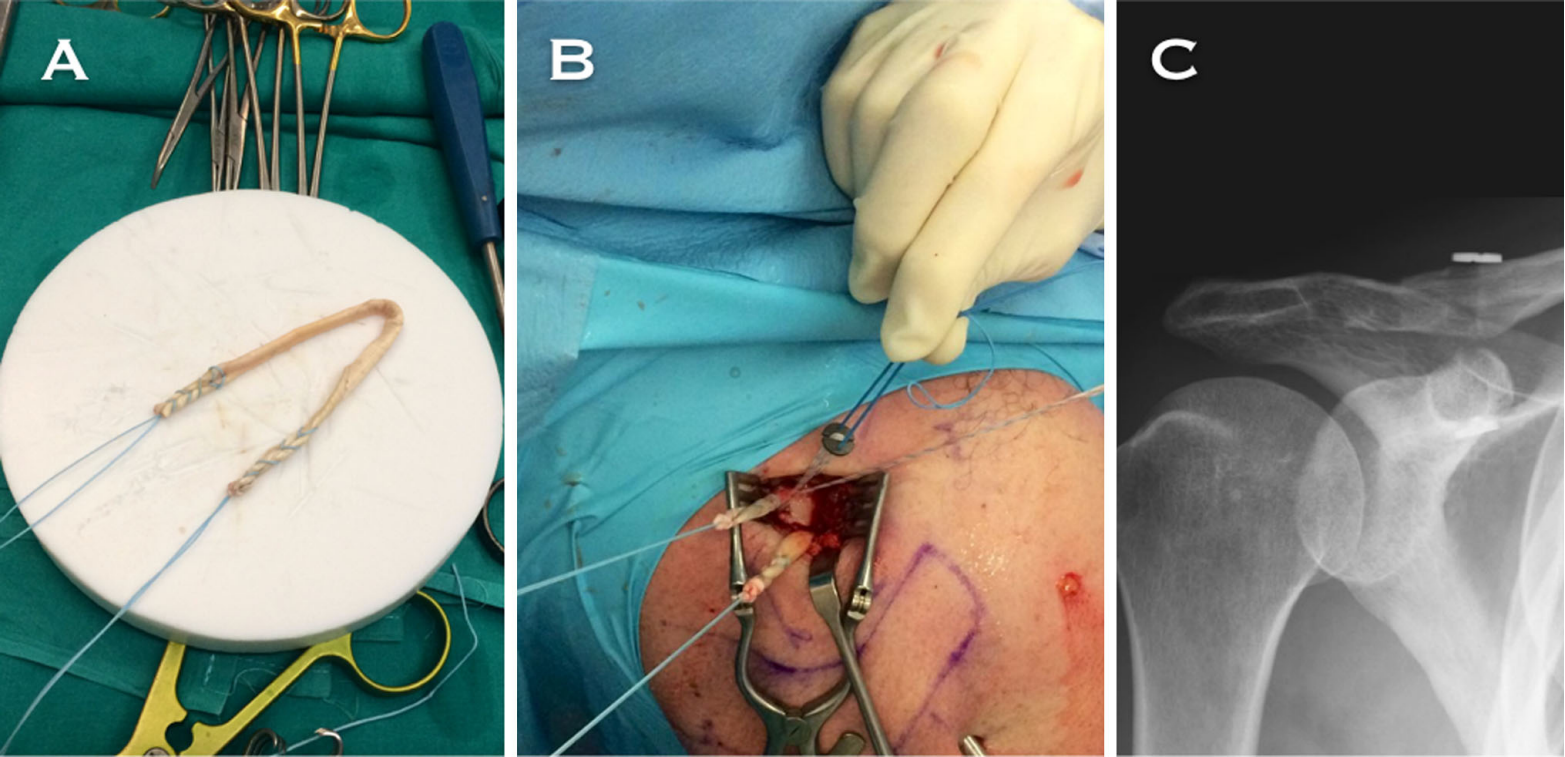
a Semitendinous allograft after being sutured with a metal-core suture in both of its limbs. b Both limbs of the graft coming out of the clavicle once fixed in both tunnels with bio-tenodesis interference screws. The ZipTight is tied by threading the sliding suture in the washer. c AP X-ray of a right shoulder in which an anatomical reconstruction of CC ligaments with tendon allograft was performed in the chronic setting. Observe the trapezoid tunnel in the clavicle, lateral to the conoid tunnel in the clavicle, through which also passes the suspension device
